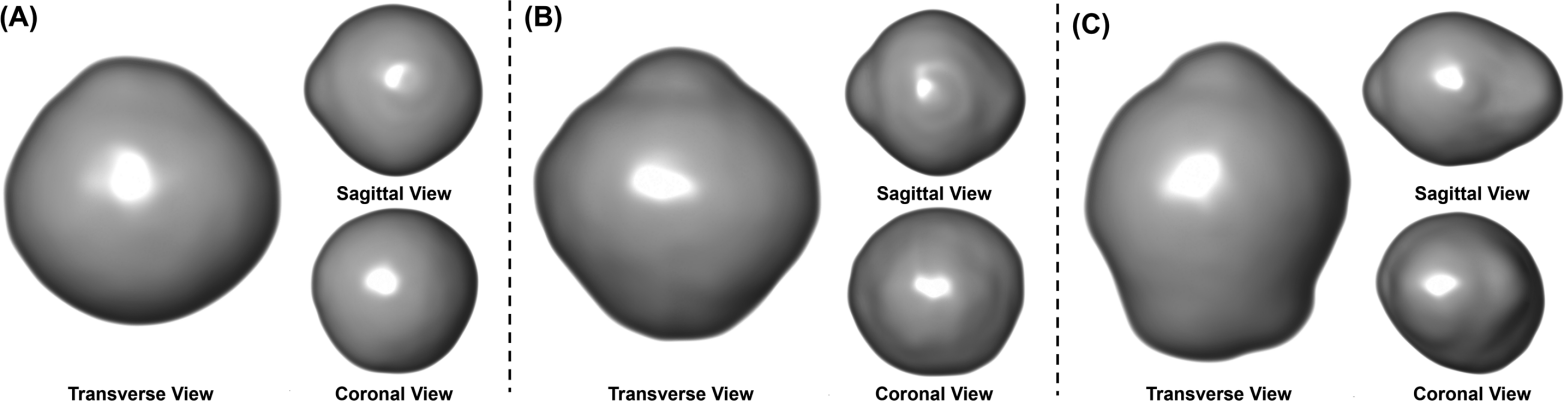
Three categories of eye shapes with three different views of the eyeball reconstructed from MRI images. This visualization was based on the segmented eyeball labels and was performed using the Volume Rendering module in the Amira software, using advanced features such as setting lighting to Specular and shade effects to Ambient. (A) Type 0 without apparent posterior globe protrusion. (B) Type 1 with elongated posterior and conical protrusion. (C) Type 2 with elongated posterior and sharp nasal or temporal protrusion.
